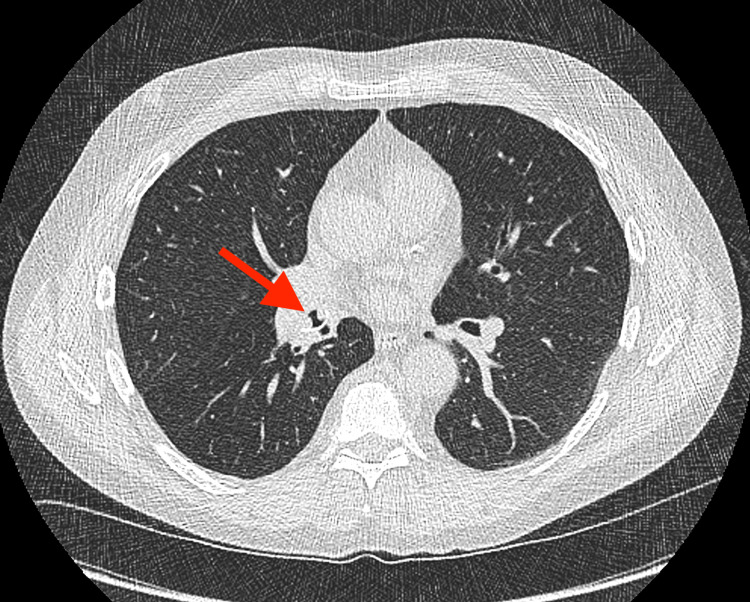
An axial image of a low-dose chest CT without intravenous contrast demonstrating a nodular, partially calcified endobronchial lesion in the right bronchus intermedius (red arrow).
